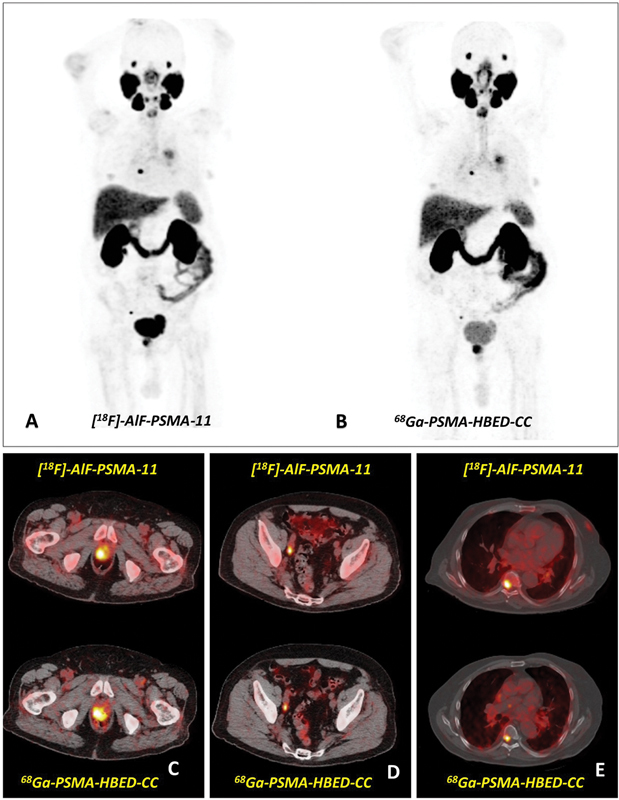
Maximum intensity projection (MIP) and axial slices of fusion positron emission tomography (PET)/computed tomography (CT) of a patient with early-stage prostate cancer scanned with (
